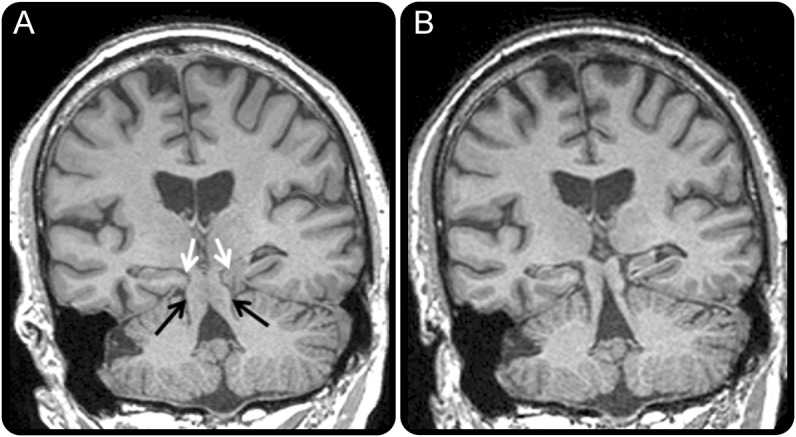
Coronal volumetric T1 MRI brain appearances of brain sagging and its resolution MRI at presentation (A) shows midbrain descent below the tentorium (black arrows) and posterior parahippocampal and lingual gyri herniation (white arrows). Follow-up MRI (B) demonstrates restoration of normal brainstem and medial temporal lobe configuration.
